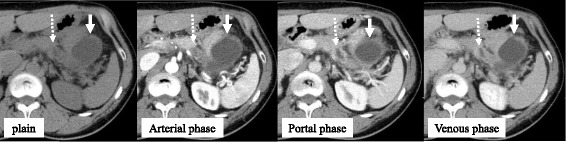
Abdominal-enhanced CT image of the pancreatic lesion. A simple cyst of the pancreatic tail was attached to the stomach, and the wall of the cyst was enhanced (*arrows*). A distal MPD was clearly dilated, but no pancreatic tumor was detectable around the stenosis of MPD (*arrow*)
